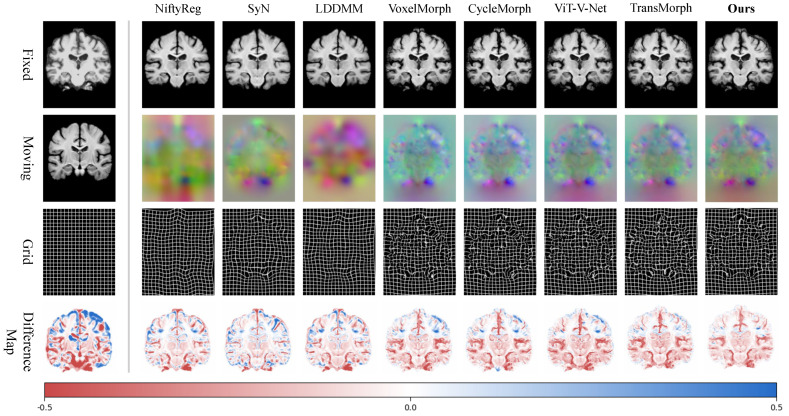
Qualitative comparison of various registration methods on the atlas-to-patient brain MRI registration task. The first column includes the fixed image, moving image, and standard grid. Excluding the first column, the first row displays the warped moving images, the second row visualizes the deformation fields (spatial dimensions x, y, z mapped to RGB color channels, respectively), the third row presents the deformed grids, and the last row shows the absolute difference maps between the warped images and the fixed image.
